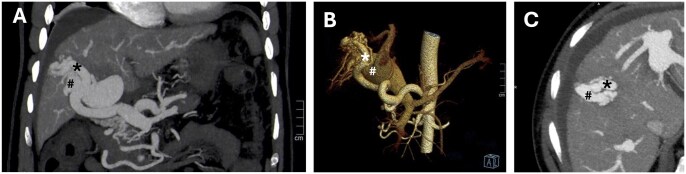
CT scan arterial phase multi-planar reconstruction (A), volume rendering (B) demonstrating intra-hepatic arterio-portal fistulas (hepatic artery branches for liver segment 8 and 5) and severe dilation of the main portal vein. Panel C shows a detailed view of the artery supplying the arteriovenous fistula and its corresponding portal drainage. The feeding artery is marked with an asterisk while the portal venous drainage is indicated by a hashtag.
